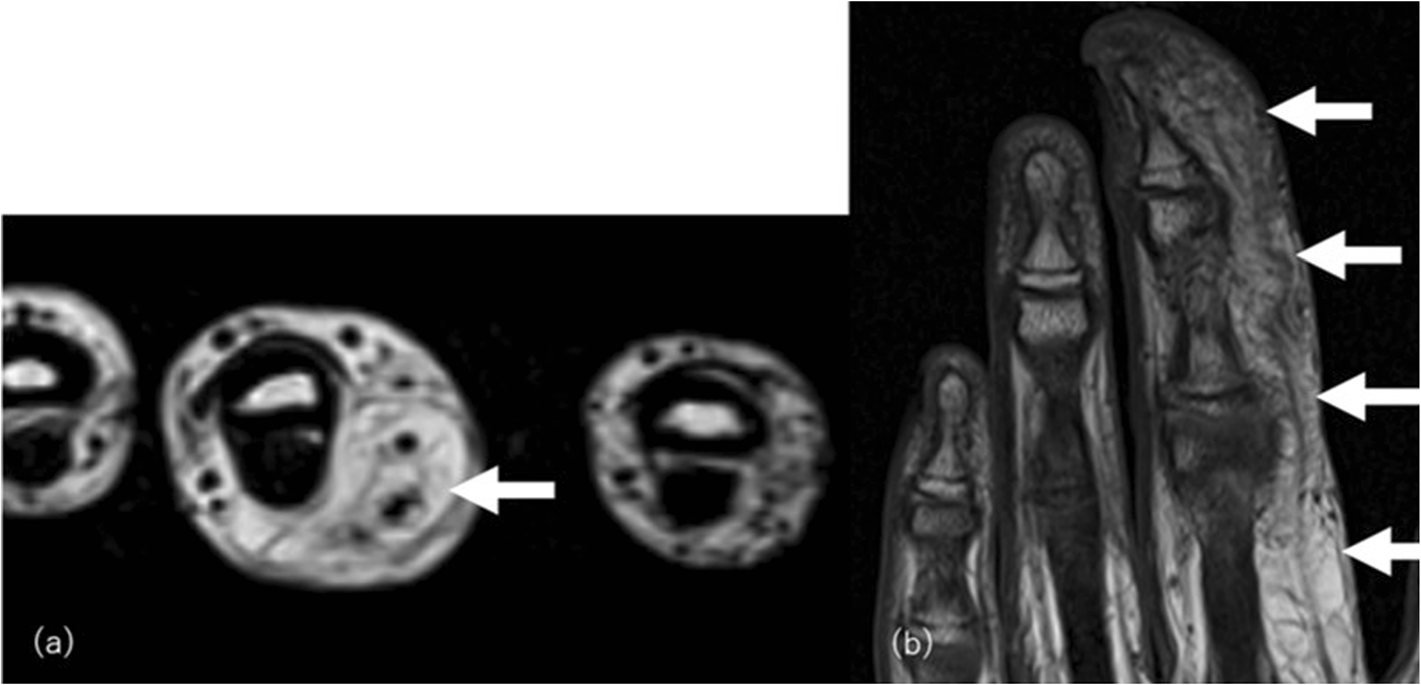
Lipomatosis of the median nerve in an 11-year-old girl without symptoms. a Axial T2- and (b) coronal T1-weighted images reveal soft-tissue hypertrophy with predominance of fat in the radial side of the middle finger along with the neurovascular structure (arrows)
